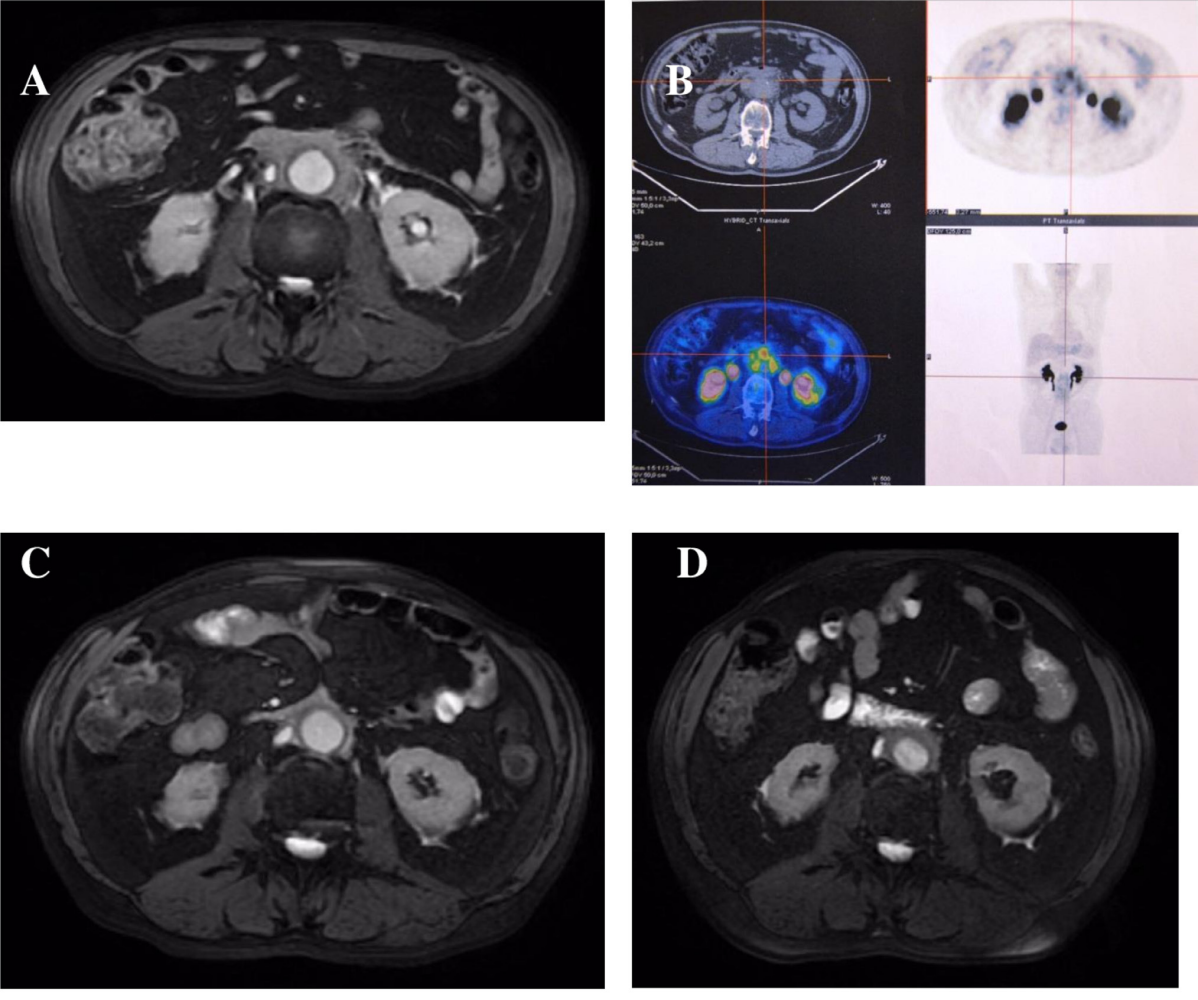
Radiologic features of patient #4 Magnetic resonance and PET/CT imagings before (A, B), 5 months (C), and 16 months (D) after treatment: (A and B) abnormal periaortic soft tissue, axial diameter 17 mm, showing high metabolic activity; (C) 60% reduction of tissue axial thickness; (D) 80% increase of periaortic mass axial diameter.
